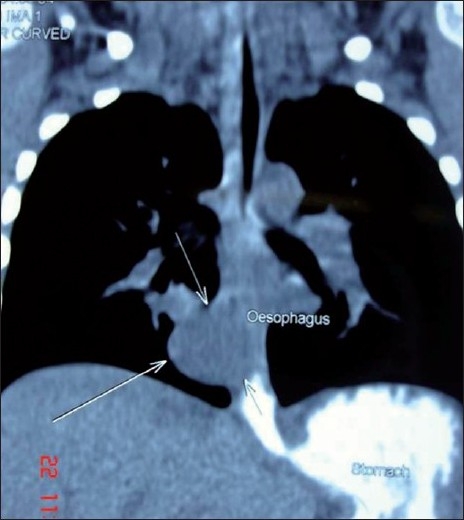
Axial reconstruction of the CT scan showing well-circumscribed cystic mass closely related to the oesophagus.
